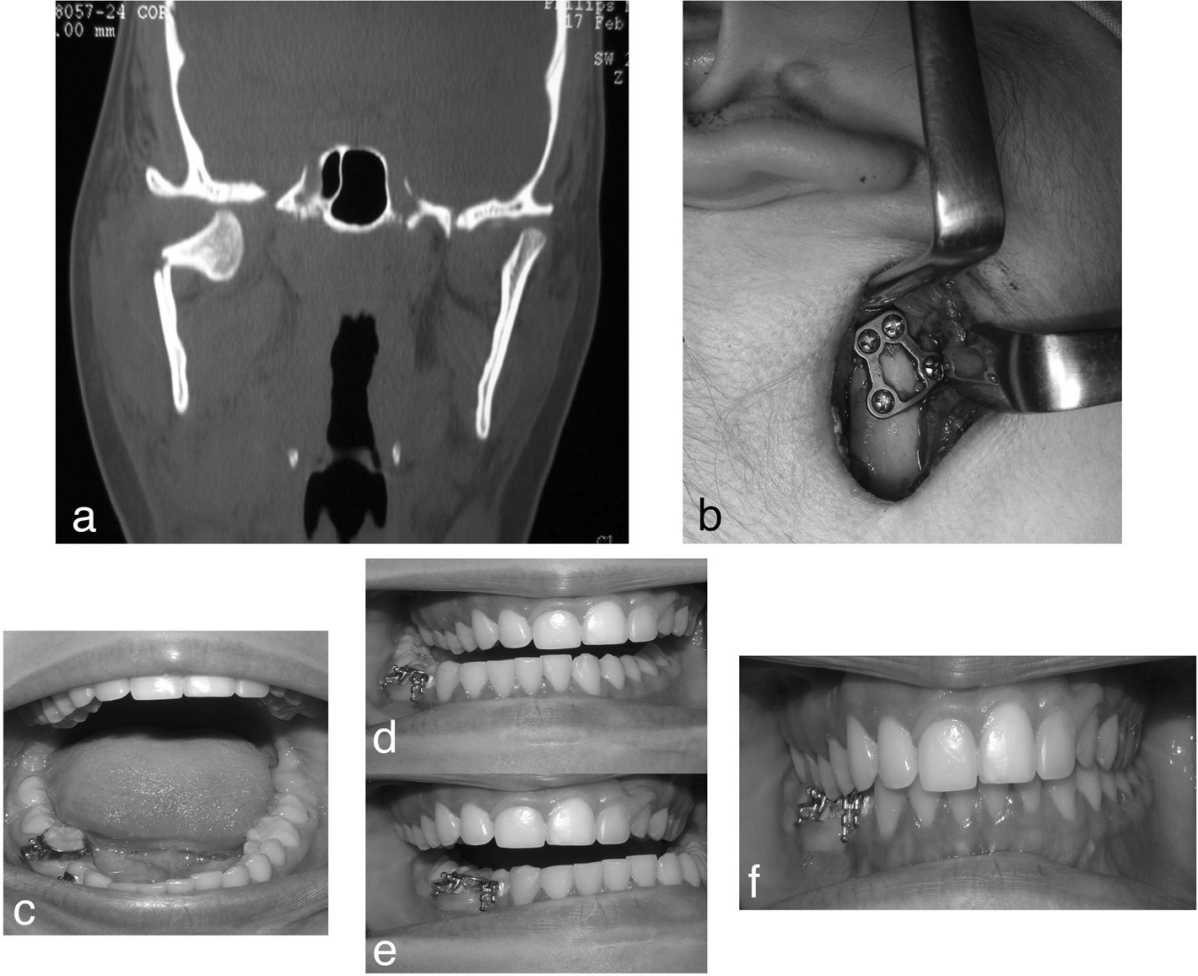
Right mandibular sub-condylar fracture: a) pre-surgery CT (coronal view); b) ORIF with a TCP plate; c) mouth opening; d) right lateral movements; e) left lateral movements; f) post-surgey occlusal plane.
